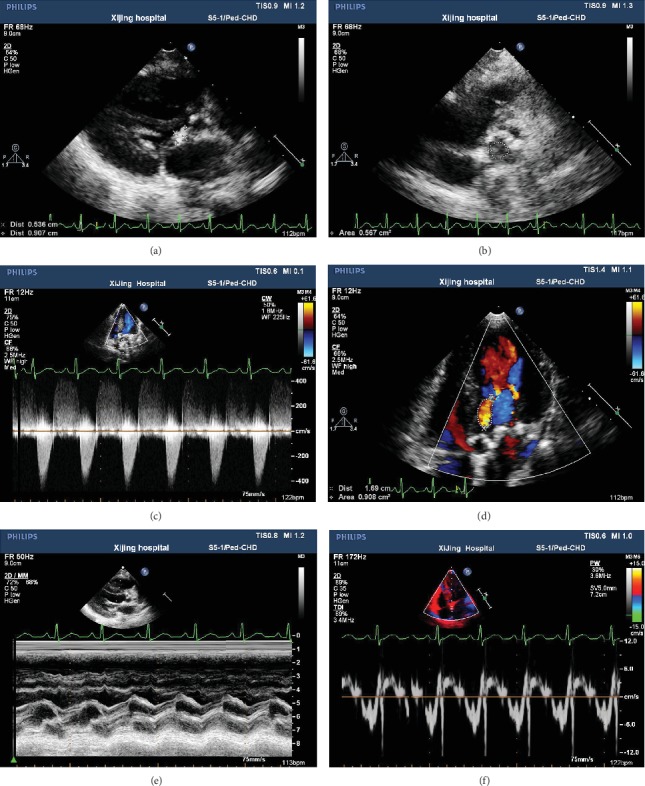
The conventional transthoracic echocardiographic examination of an elder HGPS patient (16 years old). (a) Aortic valve calcification.(b) The effective orifice area of systolic was 0.567 cm^2^. (c) The peak systolic velocity increase by color Doppler flow imaging. (d) The slight aortic valve regurgitation. (e) The left ventricular regional wall motion was normal by M-mode echocardiography. (f) The decrease in left ventricular diastole function by tissue Doppler imaging (TDI).
